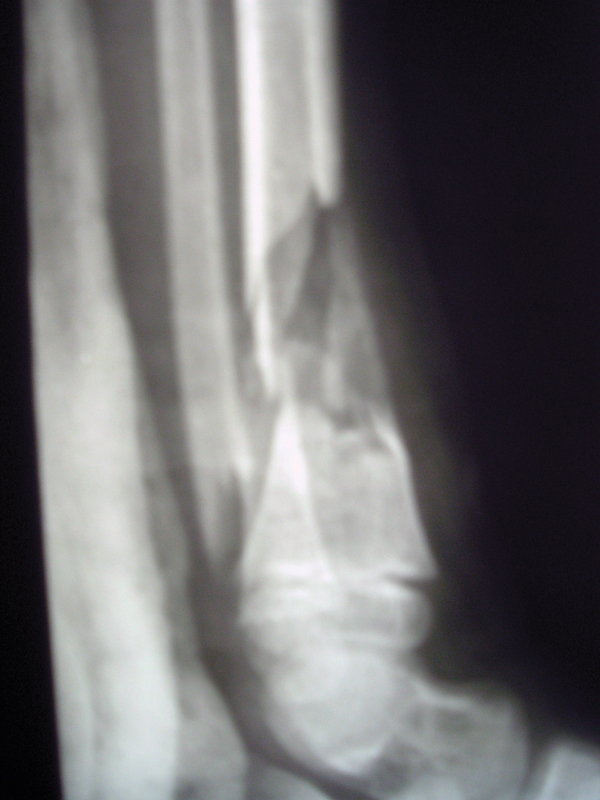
Lateral roentgenogram of the left leg demonstrates the bone defect of the distal tibia.
